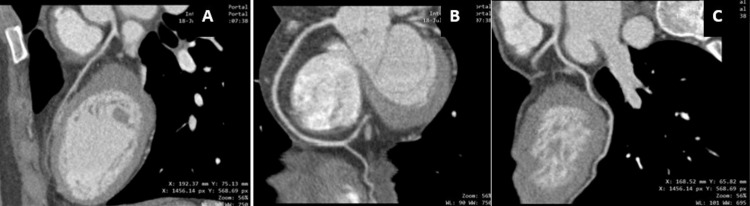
Coronary CT angiography No evidence of injury was noted. Multiplanar reconstruction image A: anterior descending artery; image B: right coronary artery; image C: circumflex artery
